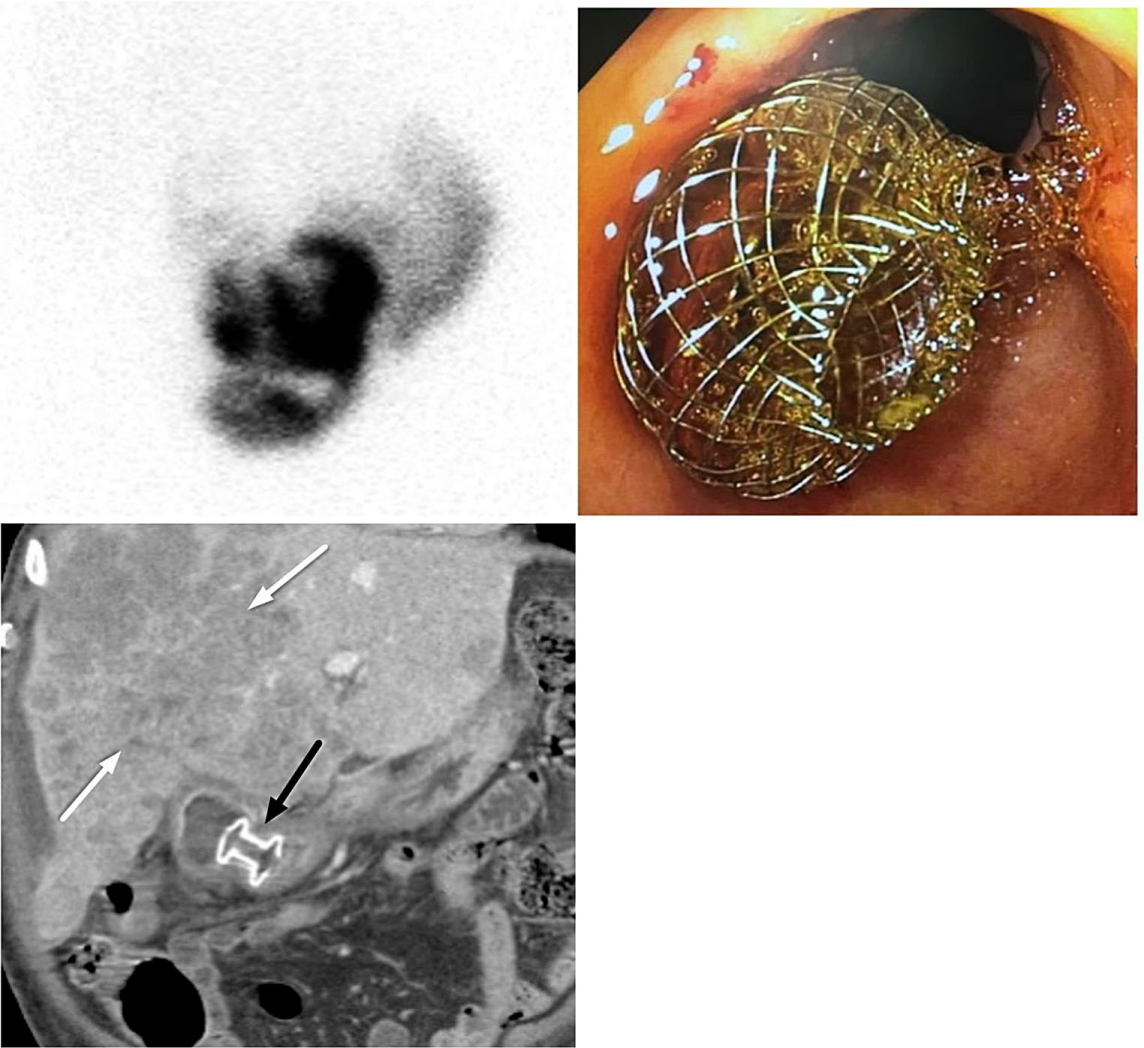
79 year-old male with prostate cancer, a poor surgical candidate, presented with acute cholecystitis and Klebsiellae bacteremia. A. HIDA scan at 4 h shows no tracer excretion into the gallbladder, diagnostic of acute cholecystitis. B. Endoscopic image from gastric antrum demonstrates LAMS cholecystogastrostomy was performed as an alternative to surgery and percutaneous cholecystostomy. C. Contrast-enhanced coronal CT image demonstrates extensive hepatic metastases (white arrows) precluding percutaneous drainage. Endoscopic cholecystogastrostomy utilizing LAMS (black arrow) was successfully performed as a therapeutic alternative
